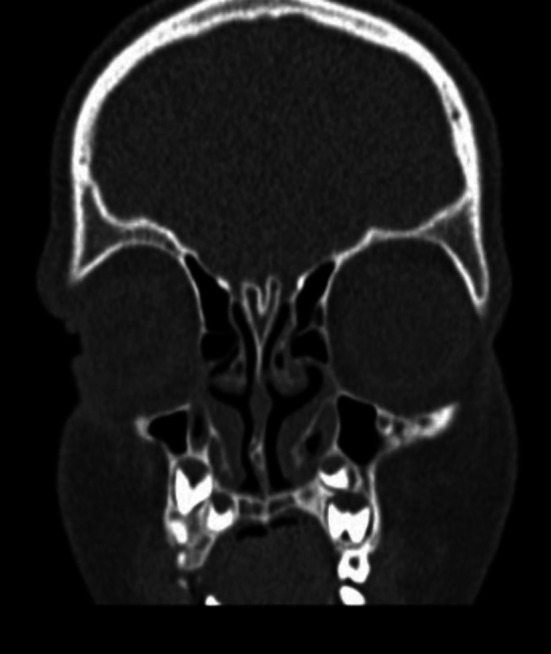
Bifid crista galli—CT coronal scan
